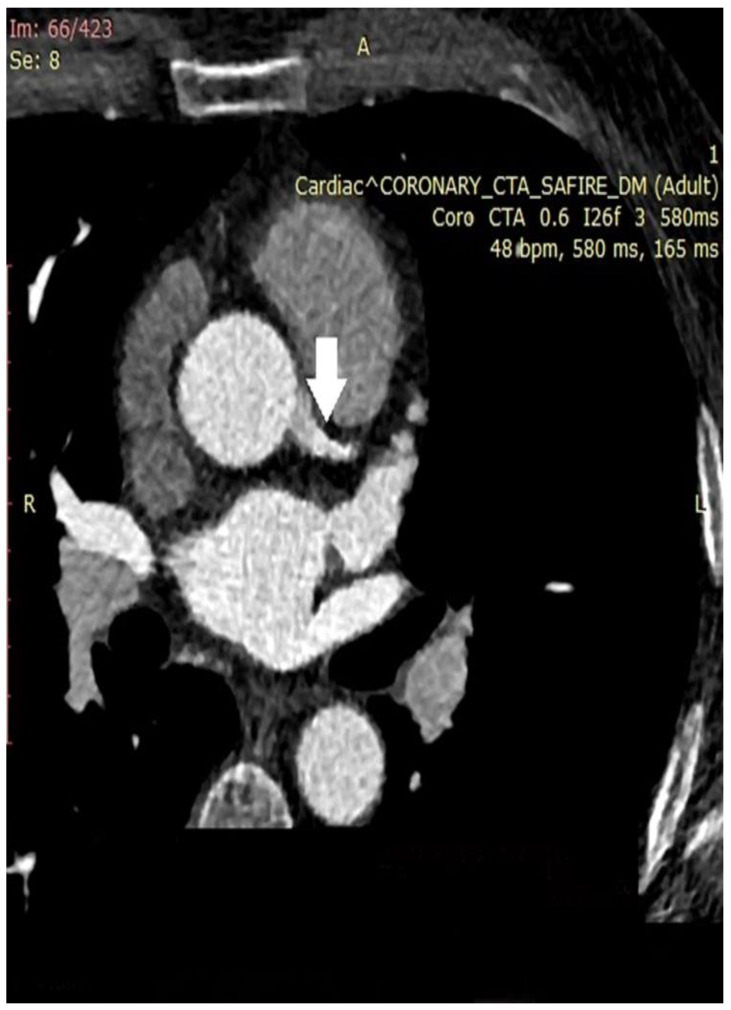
Coronary computed tomographic angiography (CCTA), axial view, and maximum intensity projection (MIP) at the level of the ascending aorta and pulmonary veins. The place of origin of the left main coronary artery is presented (white arrow). A – orientation marker indicating the position of the abdomen; R – orientation marker indicating the right side of the patient. Copyright: the authors.
